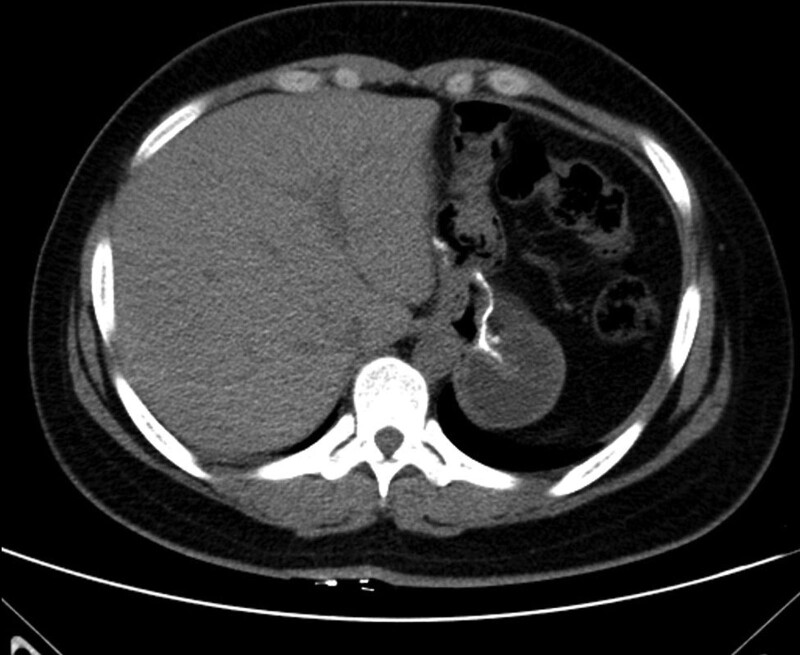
Preoperative computed tomography (CT) showed dilated gastric bursa.
